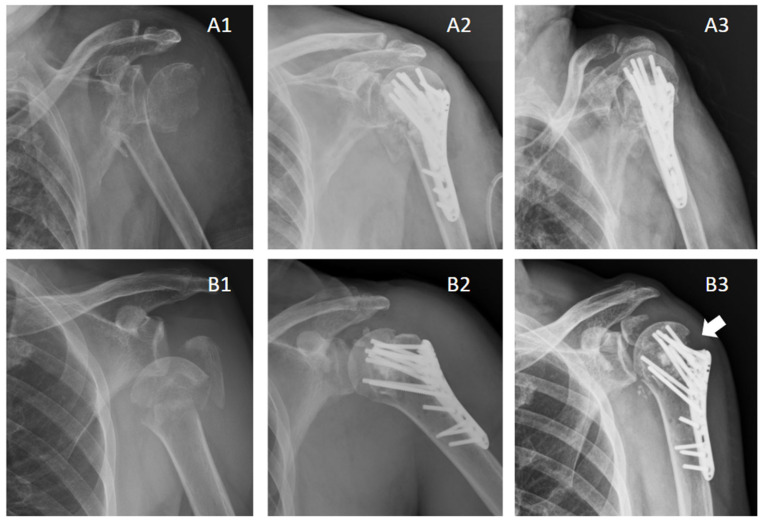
Comparison of LPF with (A1–A3) or without (B1–B3) CPBCA in elderly PHFs: Patient A: (A1) A left PHF was presented. (A2) Treated with LPF with CPBCA. (A3) Post-operative X-ray at 12 months follow-up; Patient B: (B1) A left PHF was presented. (B2) Treated with LPF without CPBCA. (B3) Post-operative X-ray at 12 months follow-up. By comparison, the patient managed with a combination of LPF and CPBCA fared better with a stable head of humerus, maintained neck–shaft angle, and position of greater tuberosity fragments; in patient B, who had LPF without CPBCA, the head of humerus had a progressive collapse, the neck–shaft angle was reduced, and greater tuberosity fragments were migrated (arrow).
